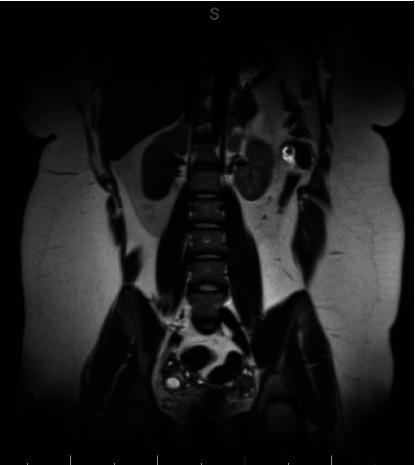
MRI abdomen/pelvis nondiagnostic for teratoma.
